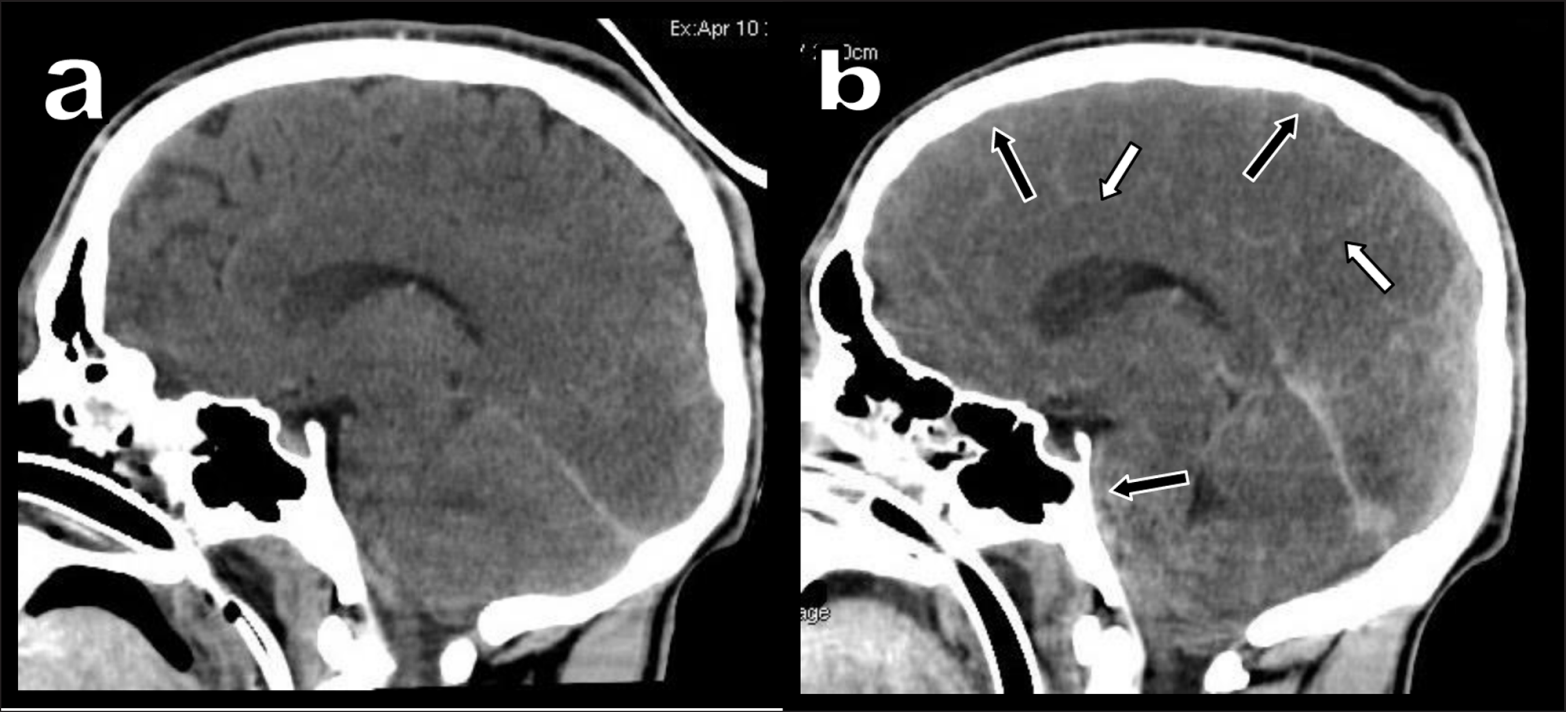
Comparison of median sagital views of unenhanced Brain CT at 5 hours (a) and 30 hours (b).
